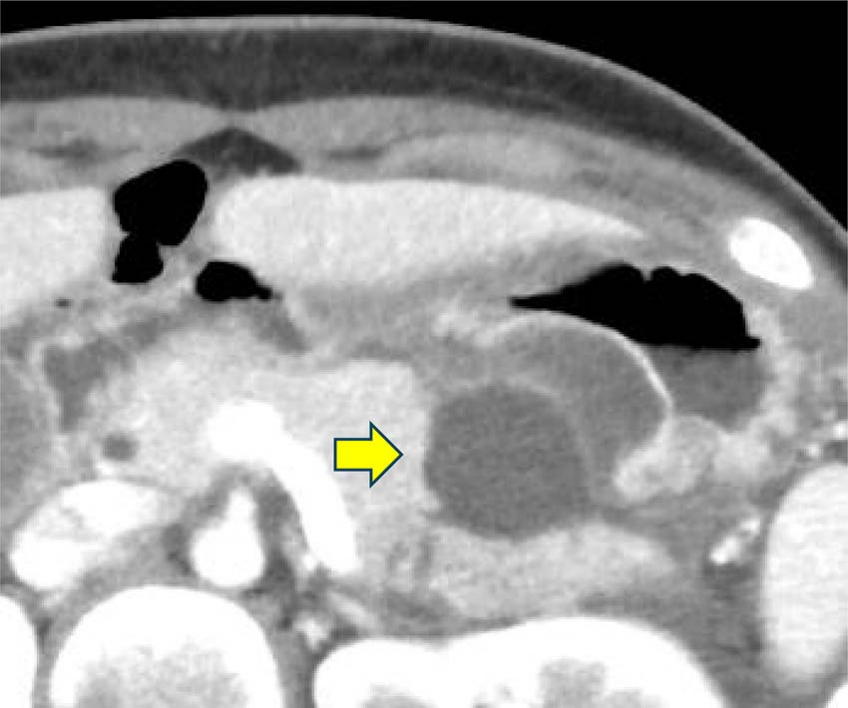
Contrast-enhanced CT of the abdomen on the fourth day. Fluid accumulation (pancreatic pseudocyst) was observed, primarily located on the ventral pancreatic body and extending toward the vicinity of the spleen, along with edematous thickening of the adjacent gastric posterior wall.
